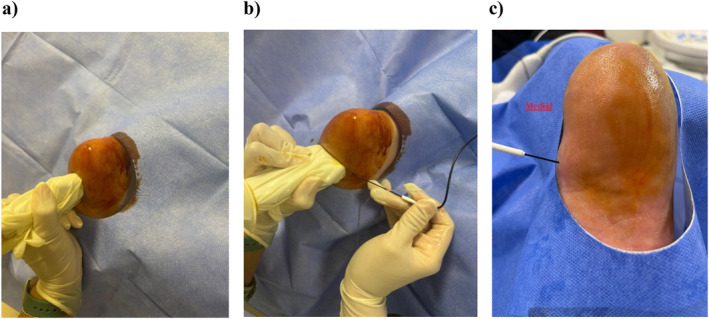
Technique of ultrasound‐guided radiofrequency ablation in plantar fasciitis. (a) Sterile preparation of the heel with the patient in the prone position and the foot dorsiflexed at 90° to optimize plantar fascia visualization. (b) Real‐time ultrasound‐guided in‐plane insertion of the RF cannula targeting the subfascial region adjacent to the medial calcaneal tubercle. (c) Final positioning of the cannula tip prior to ablation, with confirmation of proper placement on ultrasound imaging.
